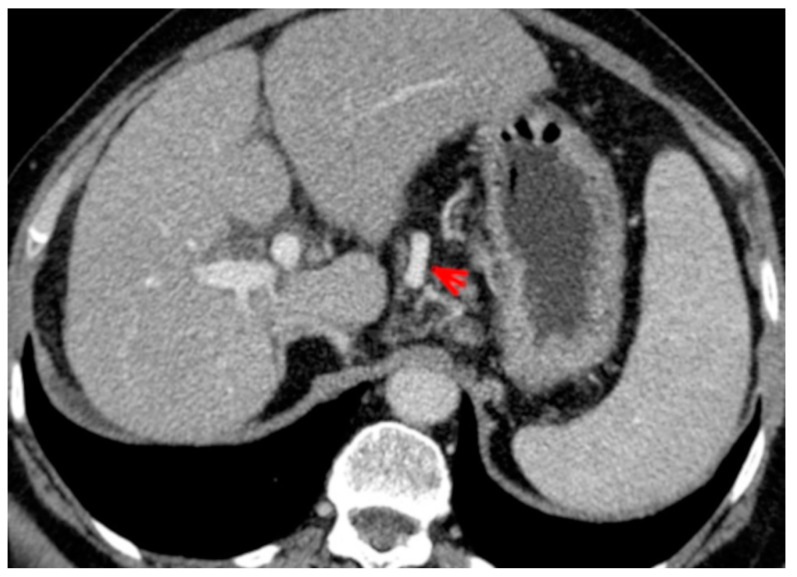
Axial contrast-enhanced CT shows enlarged left gastric vein (red arrow).
